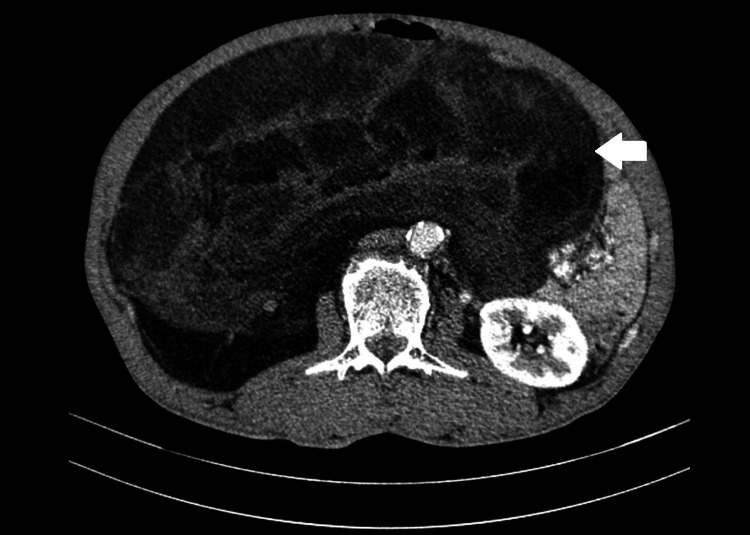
Axial plane of the abdominal CT scan revealed a large right retroperitoneal mass (white arrow)
